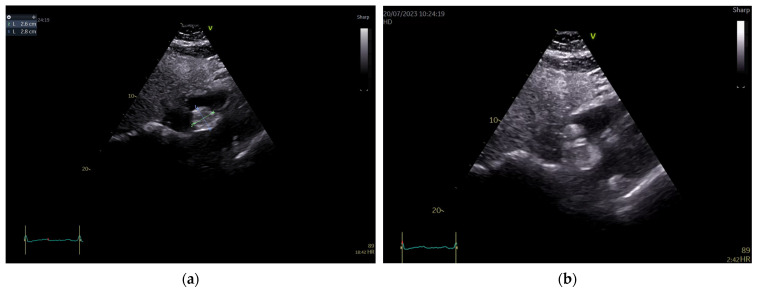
Transthoracic echocardiography, subcostal views. A round-oval, apparently homogenous mass attached to the IAS is visualized bulging in the RA. (a) Measurement of the mass revealed diameters of 26/28 mm. (b) There is no clear delineation of the RA-free walls and an infiltration at this level cannot be excluded.
